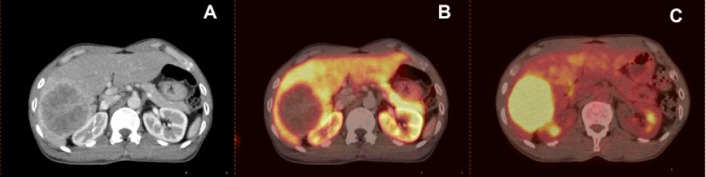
A 57-year-old man with well differentiated intrahepatic CCA. A) Transaxial contrast-enhanced CT shows a right lobe liver mass. B) Transaxial ^11^C-choline PET/CT shows no significant uptake in the lesion (T/B ratio= 0.23). C) Transaxial ^18^F-FDG PET/CT shows increased uptake in the right lobe liver mass (T/B ratio= 2.05)
